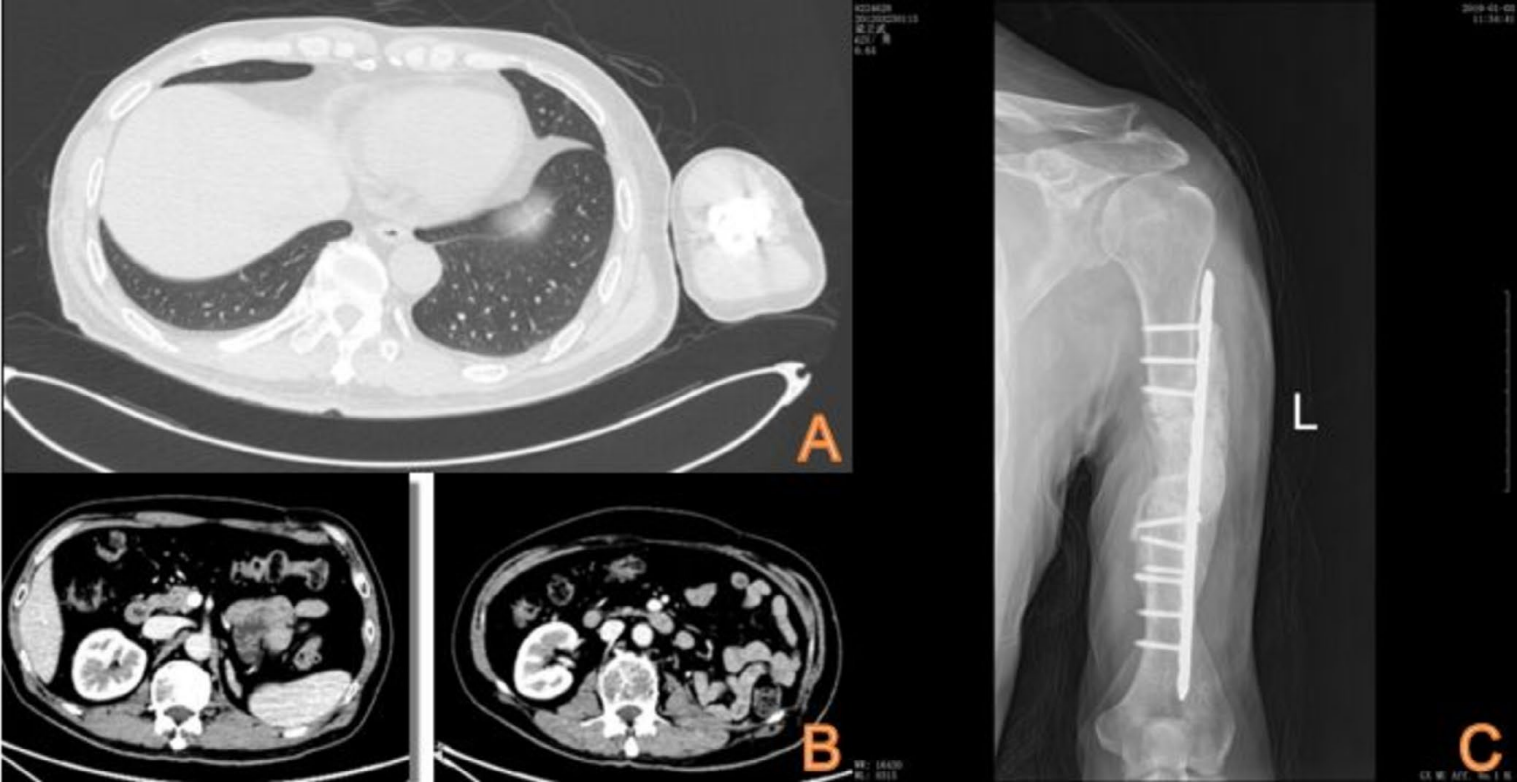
(A) Follow‐up chest CT shows significantly decreased lung metastases in May 2019. (B) Follow‐up urinary CT reveals decreased size of left adrenal metastasis in March 2018. (C) Left humerus X‐ray review shows no local bone metastatic recurrence and good humerus fixation in January 2019.
